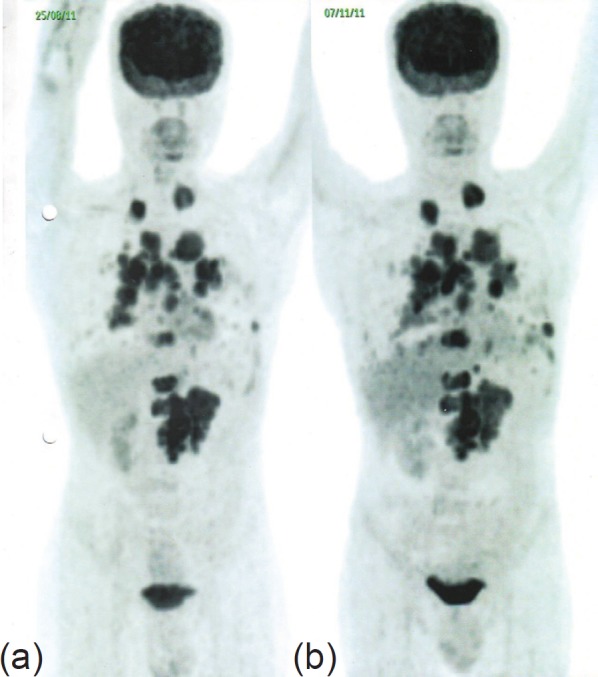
PET-CT in November 2011 (b) shows ~50–100% increase in the size of the pulmonary lesions, ~15% increase in the size of the LRF mass, and appearance of new pleural deposits compared to PET-CT in August 2011 (a), indicating disease progression.
